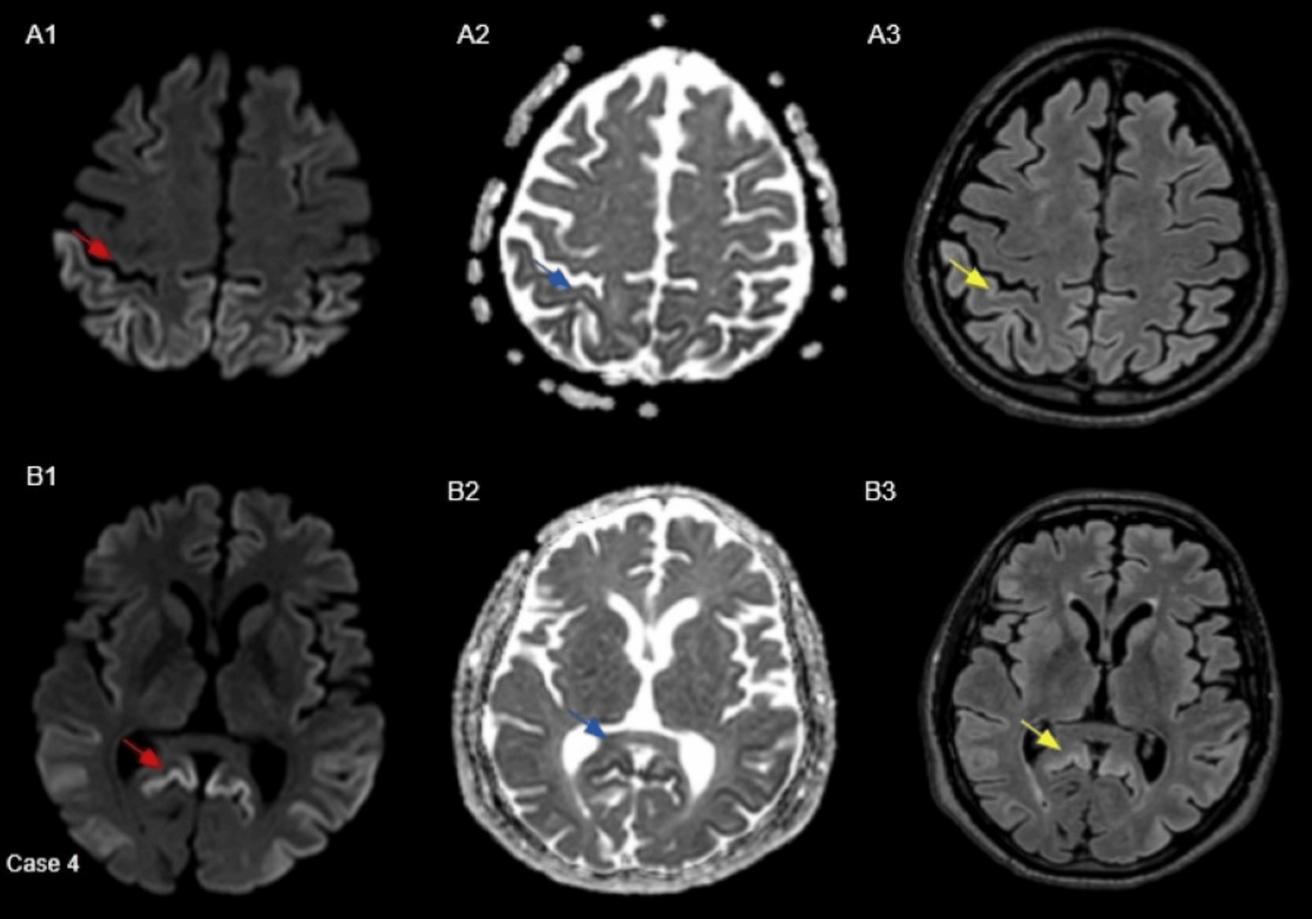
Axial B-1000 diffusion images (red arrows in A1, B1) demonstrate cortical hyperintensity involving the bilateral parieto-occipital lobe convexities, more pronounced on the right. Corresponding ADC maps (blue arrows in A2, B2) show reduced signal in the same regions, and FLAIR images (yellow arrows in A3, B3) reveal cortical hyperintensity. The basal ganglia, thalami, and cerebellum were unremarkable. ADC: apparent diffusion coefficient; FLAIR: fluid-attenuated inversion recovery
